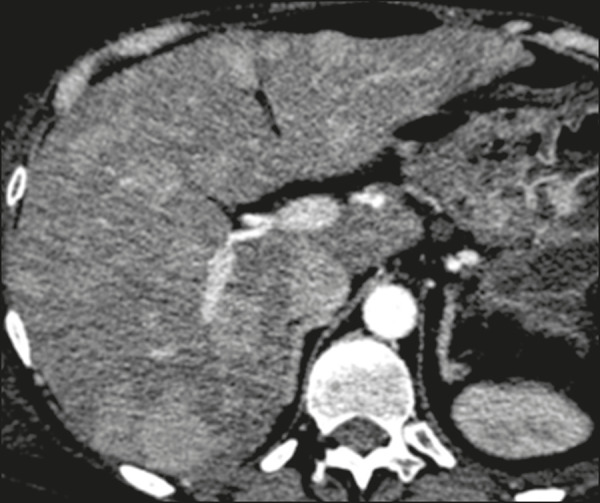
Arterial phase CT scan showing an NEN metastasis characterized by multiple liver nodules with a hypervascular enhancement pattern.
